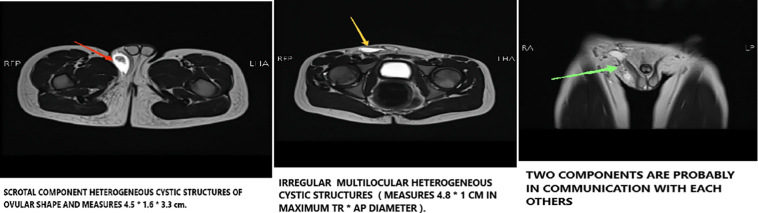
MRI showing oval-shaped and multilocular lesion, measuring 4.5 × 1.6 × 3.3 cm (CC, TR, and AP dimensions, respectively).
